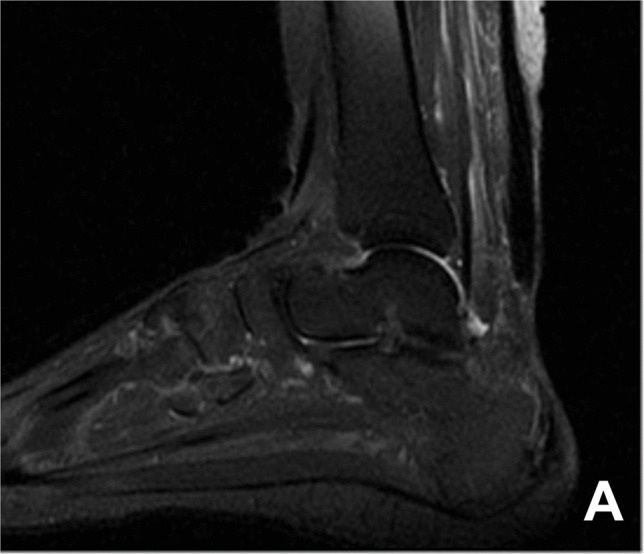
A 52-year-old female patient, presented with preoperative MRI images showing a complete rupture of the Achilles tendon at the insertion point
